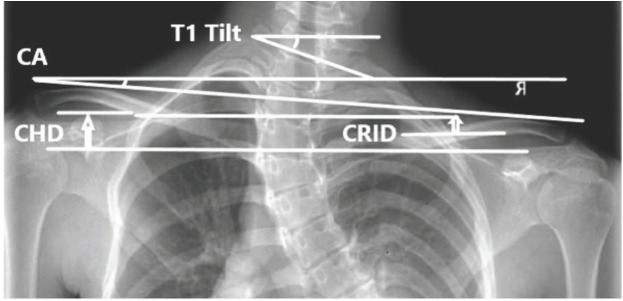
Radiographic parameters of neck-shoulder balance (CA: clavicle angle, CHD: coracoid height difference, CRID: clavicle rib intersection distance).
